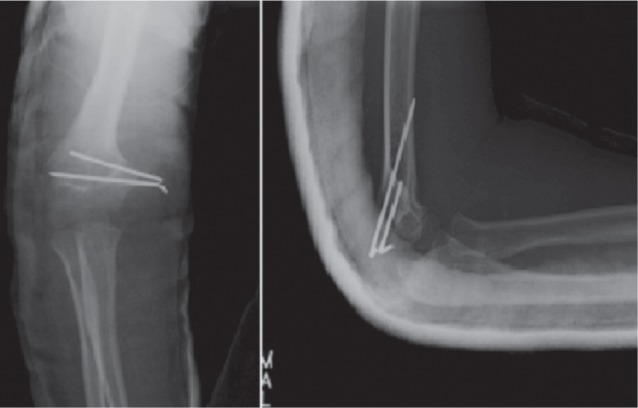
Post operative radiographs of the elbow after pinning of the medial condyle and immobilization of the joint with a plaster splint.
